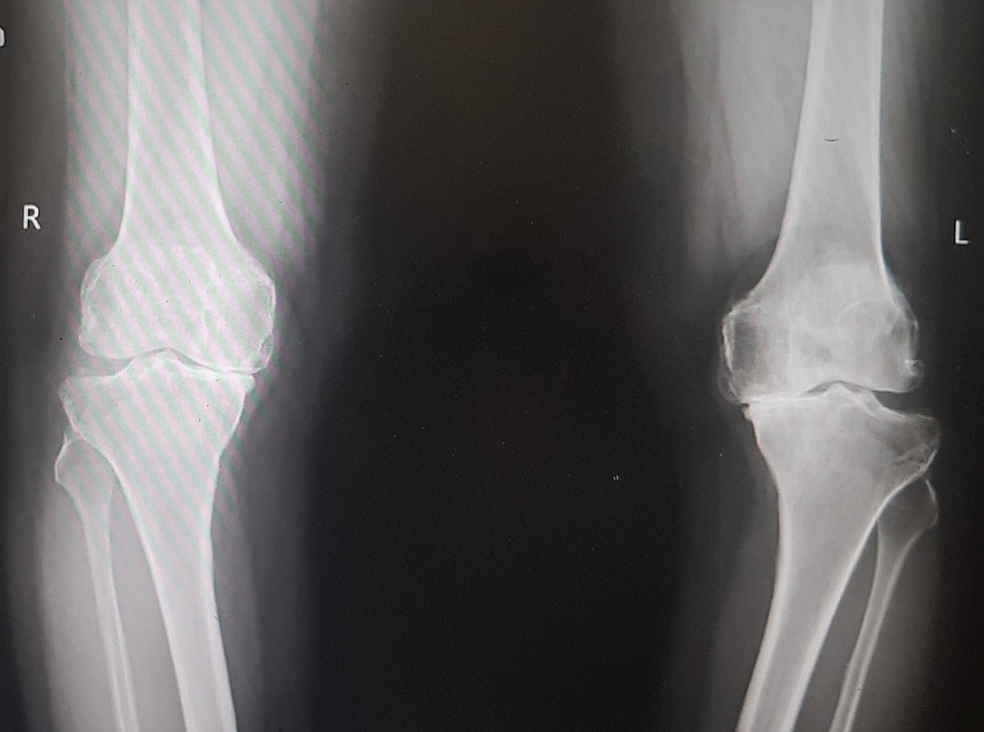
Bilateral knee radiographs (anteroposterior standing view) shows severe osteoarthritis changes
